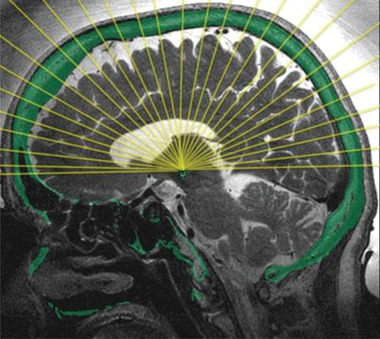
Principle of a helmet system with 1024 ultrasound transducer elements for focal surgery or focal blood–brain barrier (BBB) opening. Reproduced with permission. Copyright INSIGHTEC (www.insightec.com).
